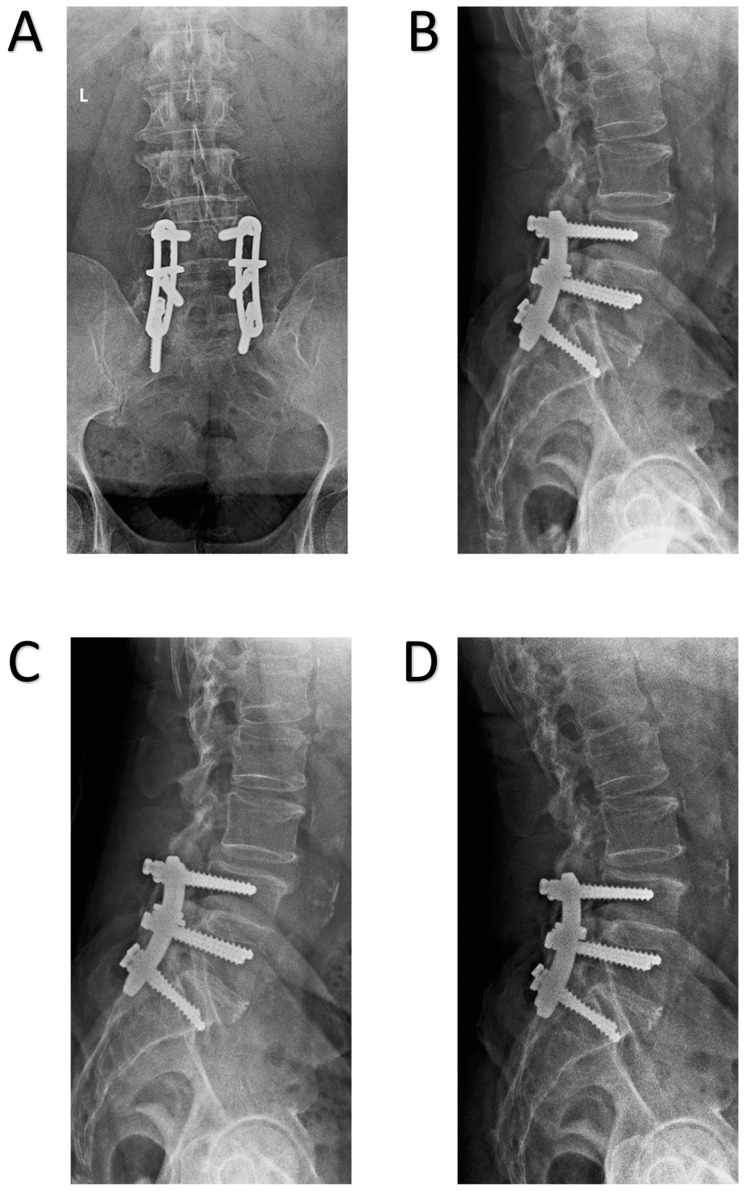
Upright AP (A), lateral (B), lateral flexion (C), and lateral extension (D) radiographs of the lumbar spine on presentation revealed intact Luque implants at L4-S1 with mobile Grade 1 degenerative anterolisthesis at L3-4 AP: anteroposterior
